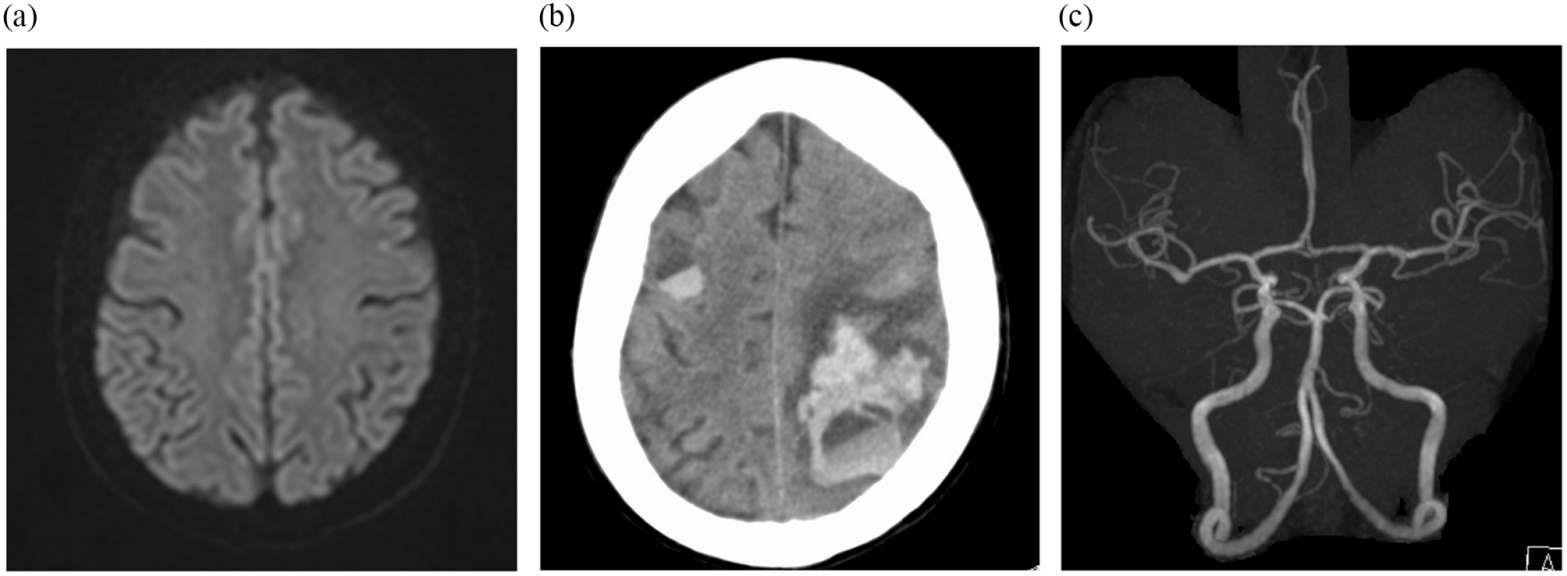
(a) Preoperative brain magnetic resonance imaging of a patient showing almost normal. (b) Postoperative brain computed tomography image of a patient showing broad cerebral hemorrhage. (c) Preoperative brain magnetic resonance angiography of a patient showing no mycotic aneurysm
